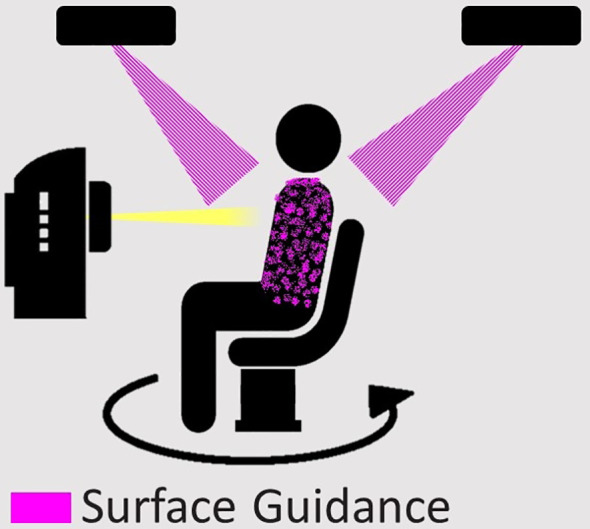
• In-room patient setup: pose and isocenter alignment • Planar and volumetric imaging for tumour targeting • Continuous patient position monitoring	• Laser/surface guidance systems adapted for an upright position • Upright 2D/3D/4D on-board imaging using kV/MV photons or MRI • Online position monitoring adapted for a continuously moving patient
